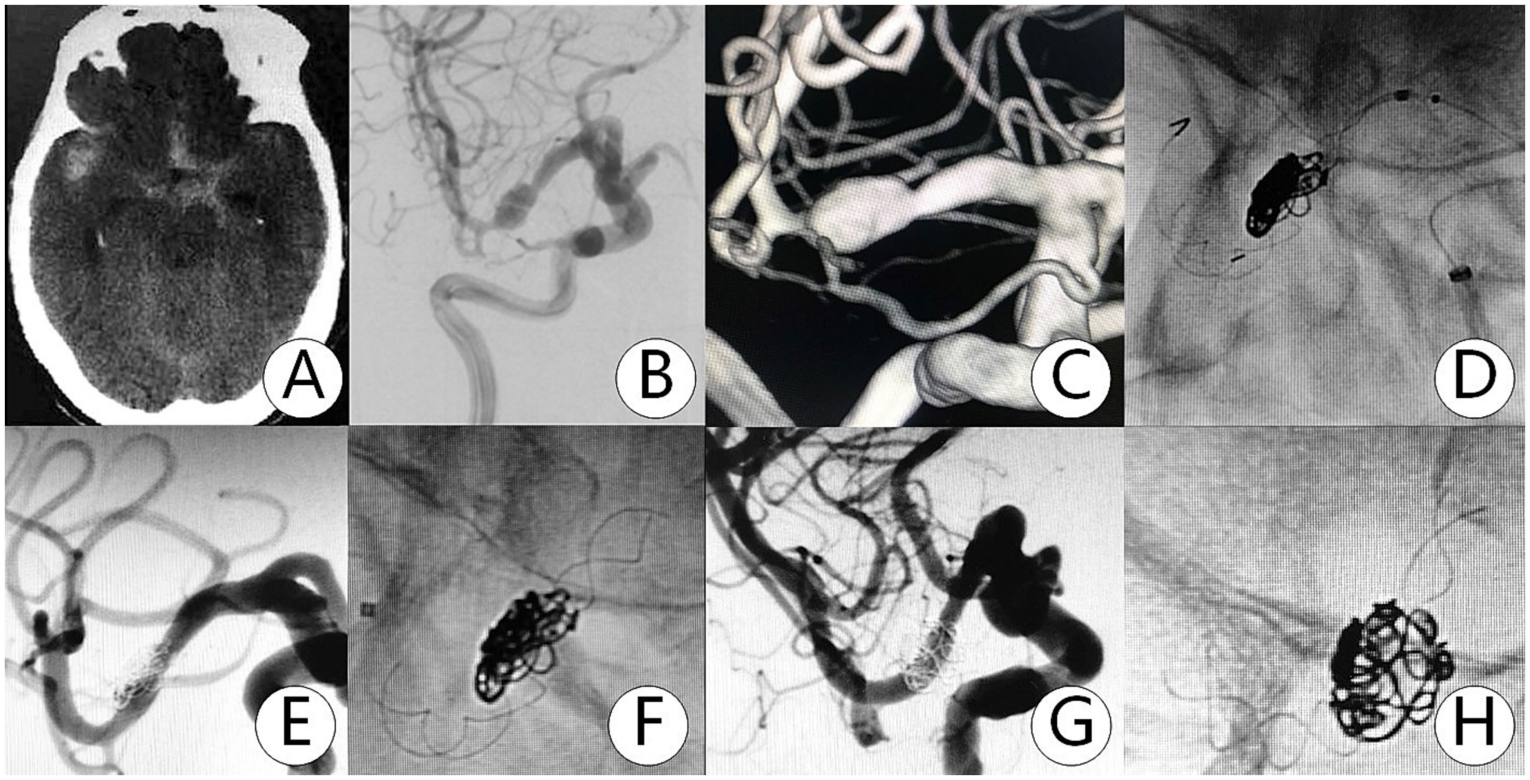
Patient experienced a subarachnoid hemorrhage with a Hunt–Hess grade I for 5 h. (A) CT showed subarachnoid hemorrhage in the Sylvian cistern and pericallosal cistern. (B,C) DSA revealed a dissecting aneurysm in the M1 segment. (D–F) The TFD (3.0×25 mm) and coils were successfully deployed in the aneurysm. (G,H) DSA at 13 months follow-up showed that the dissecting aneurysm was completely embolized.
